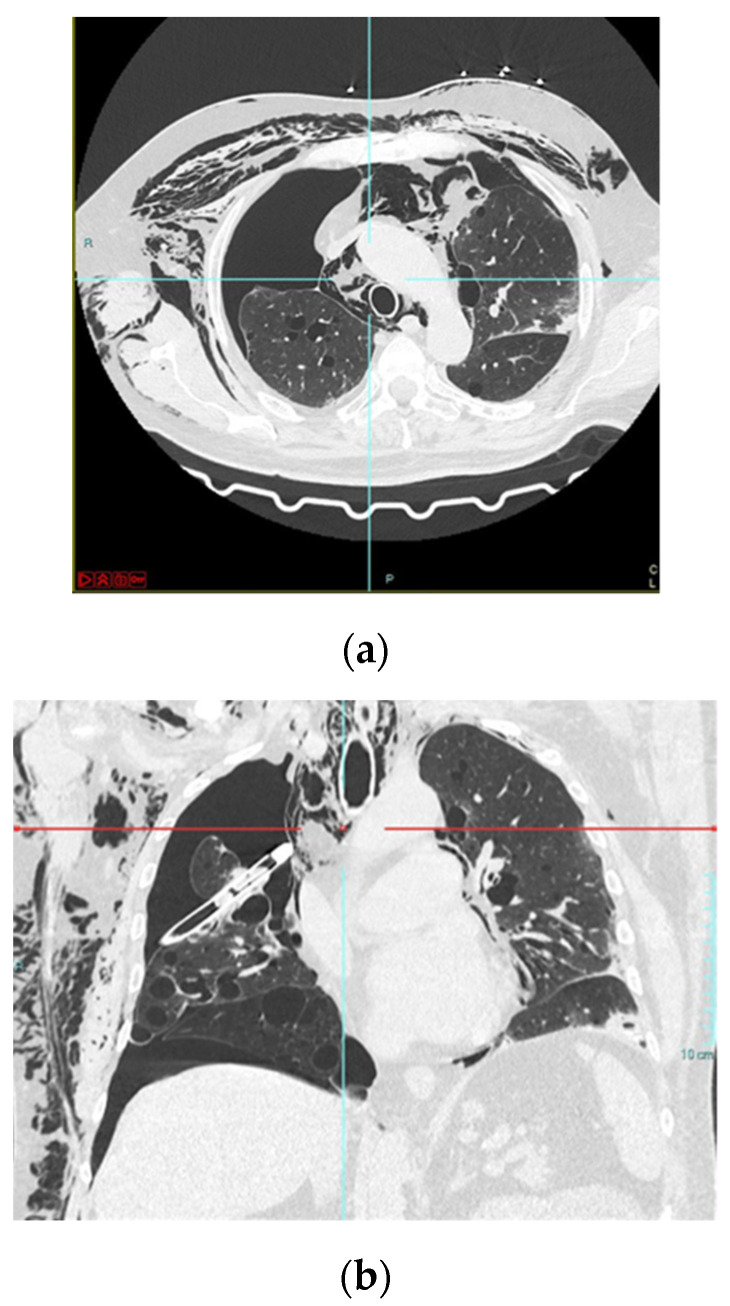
A typical case of a COVID-19 pneumothorax and pneumomediastinum patient (a) chest TC showing pneumothorax extended at 75% of the right lung and large mediastinal emphysema; (b) Coronal CT showing chest drainage for pneumothorax at right lung anteroposterior projection image.
